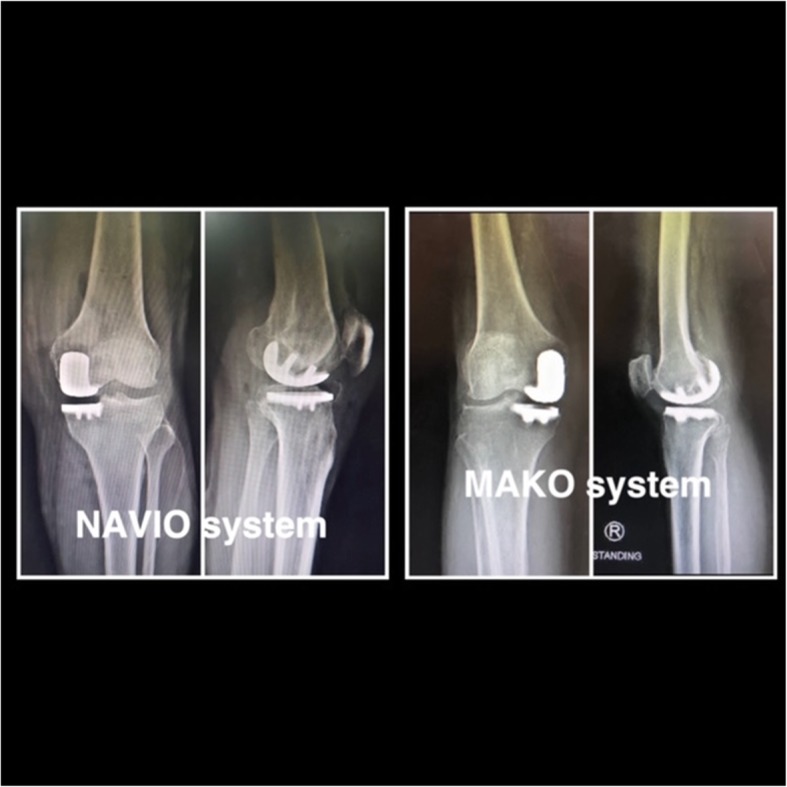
X-rays of postoperative patient (our series) who underwent unicompartmental knee arthroplasty with image-based MAKO system and imageless NAVIO system. This picture show images of two patients who underwent two different procedure
